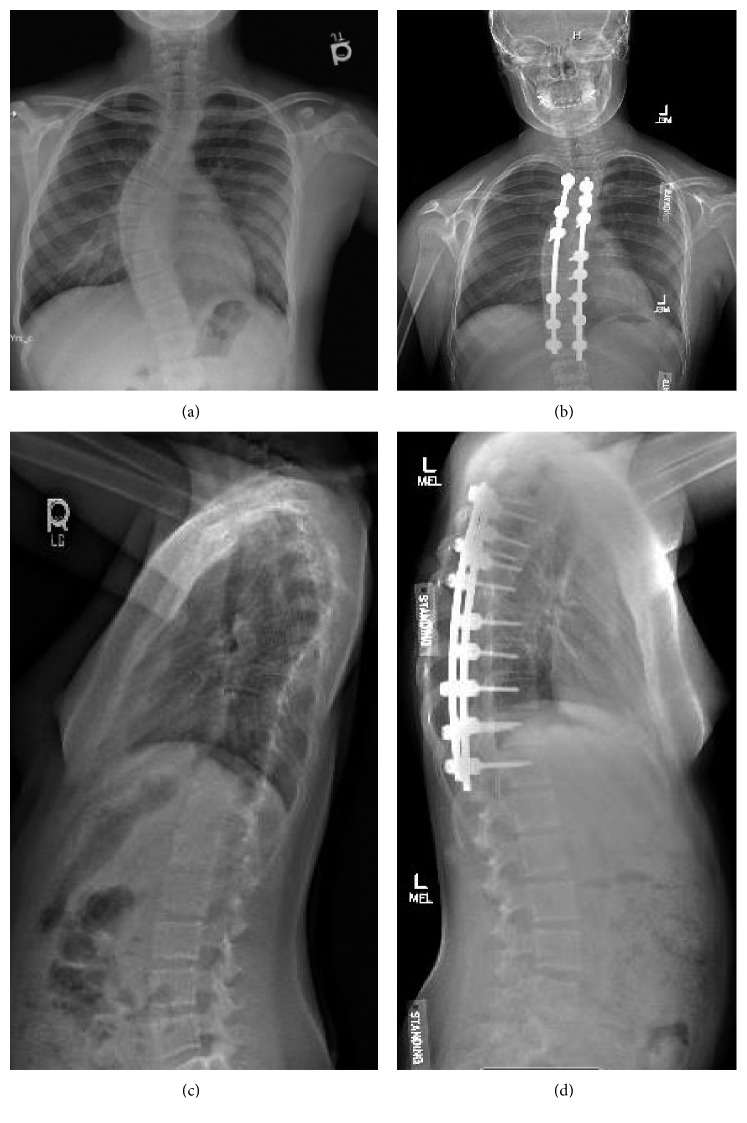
(a, c) Chest radiograph from admission for pulmonary exacerbation, October 4, 2016. (b, d) Spinal series six months after posterior spinal fusion, June 29, 2017.
